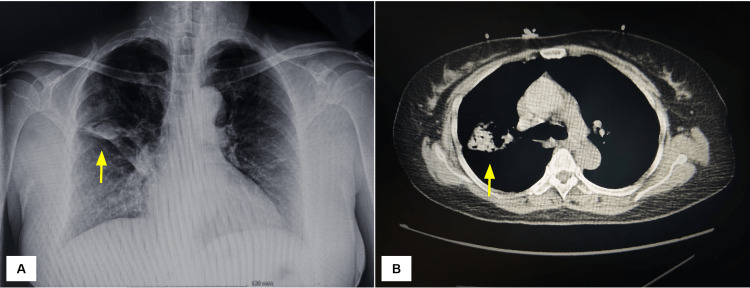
Chest imaging findings (A) Anterior-posterior chest radiograph showing a focal density in the right upper lobe with adjacent linear scarring/atelectasis and (B) CT scan of the chest showing a partial consolidation of the right anterior and posterior segments of the right upper lobe. CT: computed tomography.
